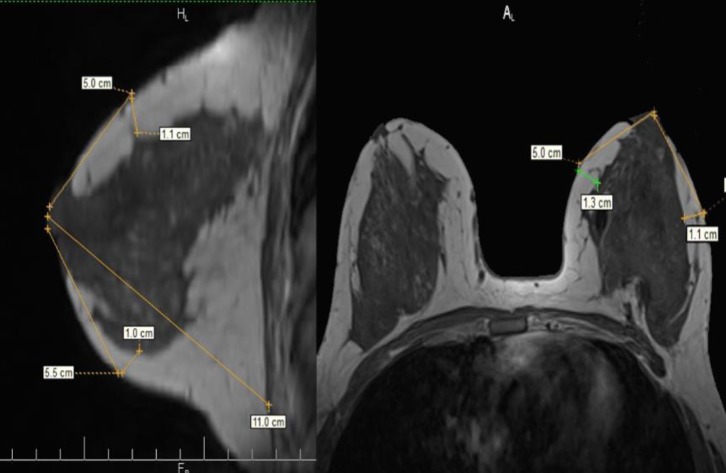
Preoperative breast MRI in T1 sequence without fat saturation. Measurements of the subcutaneous layer in the established points in sagittal and transverse sections.
